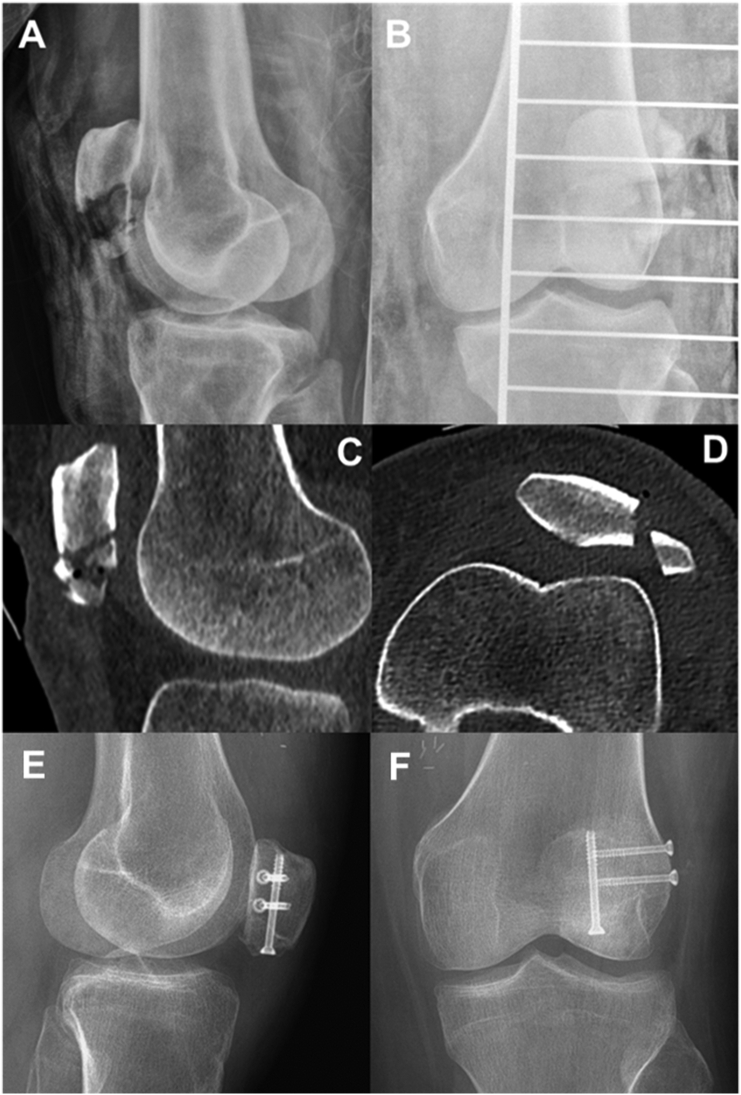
Preoperative and postoperative imaging of the original injury. A: Preoperative lateral X-ray of the knee. B: Preoperative AP X-ray of the knee. C: Preoperative sagittal CT of the knee. Important comminution is visible at the distal end of the patella. D: Preoperative axial CT of the knee. A displaced vertical fracture of the lateral facet is visible. E: Postoperative lateral X-ray of the knee at the fifth month of follow-up. Notice complete fracture healing and normal patellar height. F: Postoperative AP X-ray of the knee at the fifth month of follow-up.
